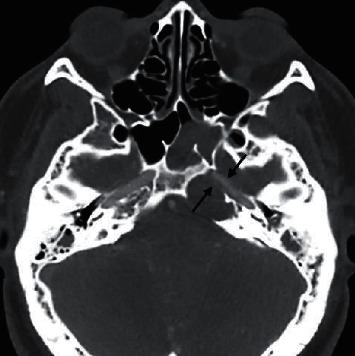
CT angiogram: the left carotid canal wall is dehiscent, and the ICA is narrowed (arrows), presumably due to vasospasm from adjacent inflammation. CTA is valuable to confirm that an expansile petrous apex lesion is not an ICA aneurysm.
